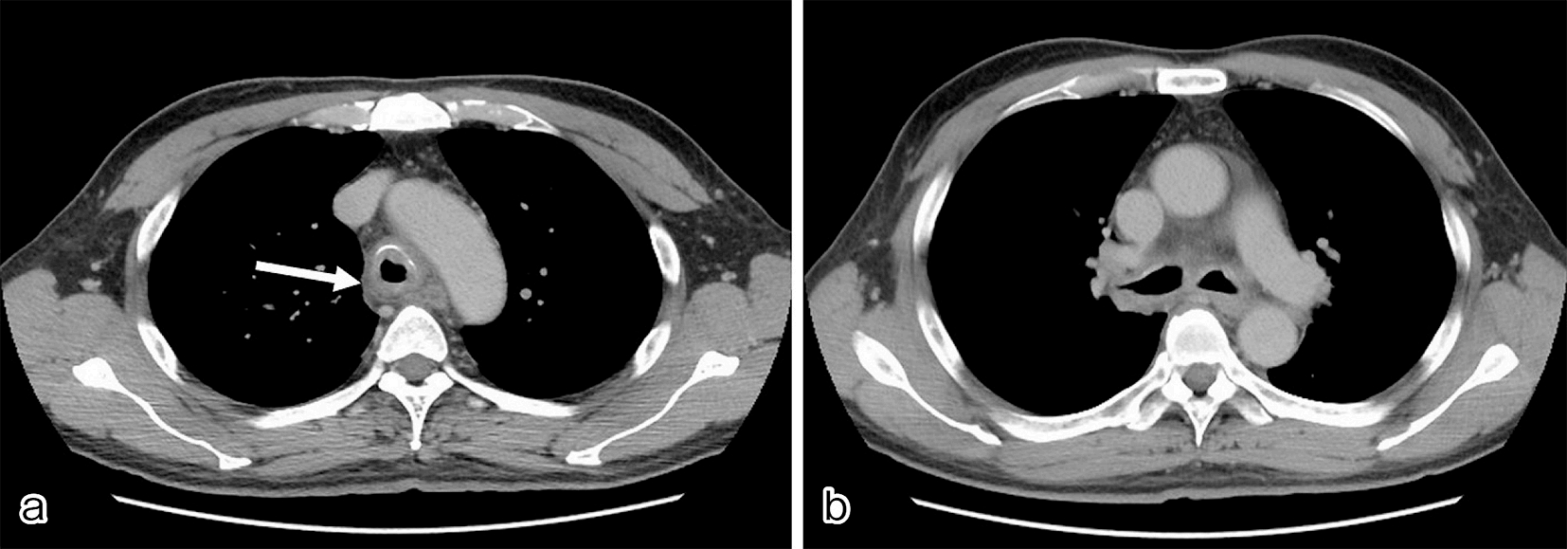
Contrast-enhanced computed tomography revealed thickening of the tracheal and bilateral bronchial tube walls.
